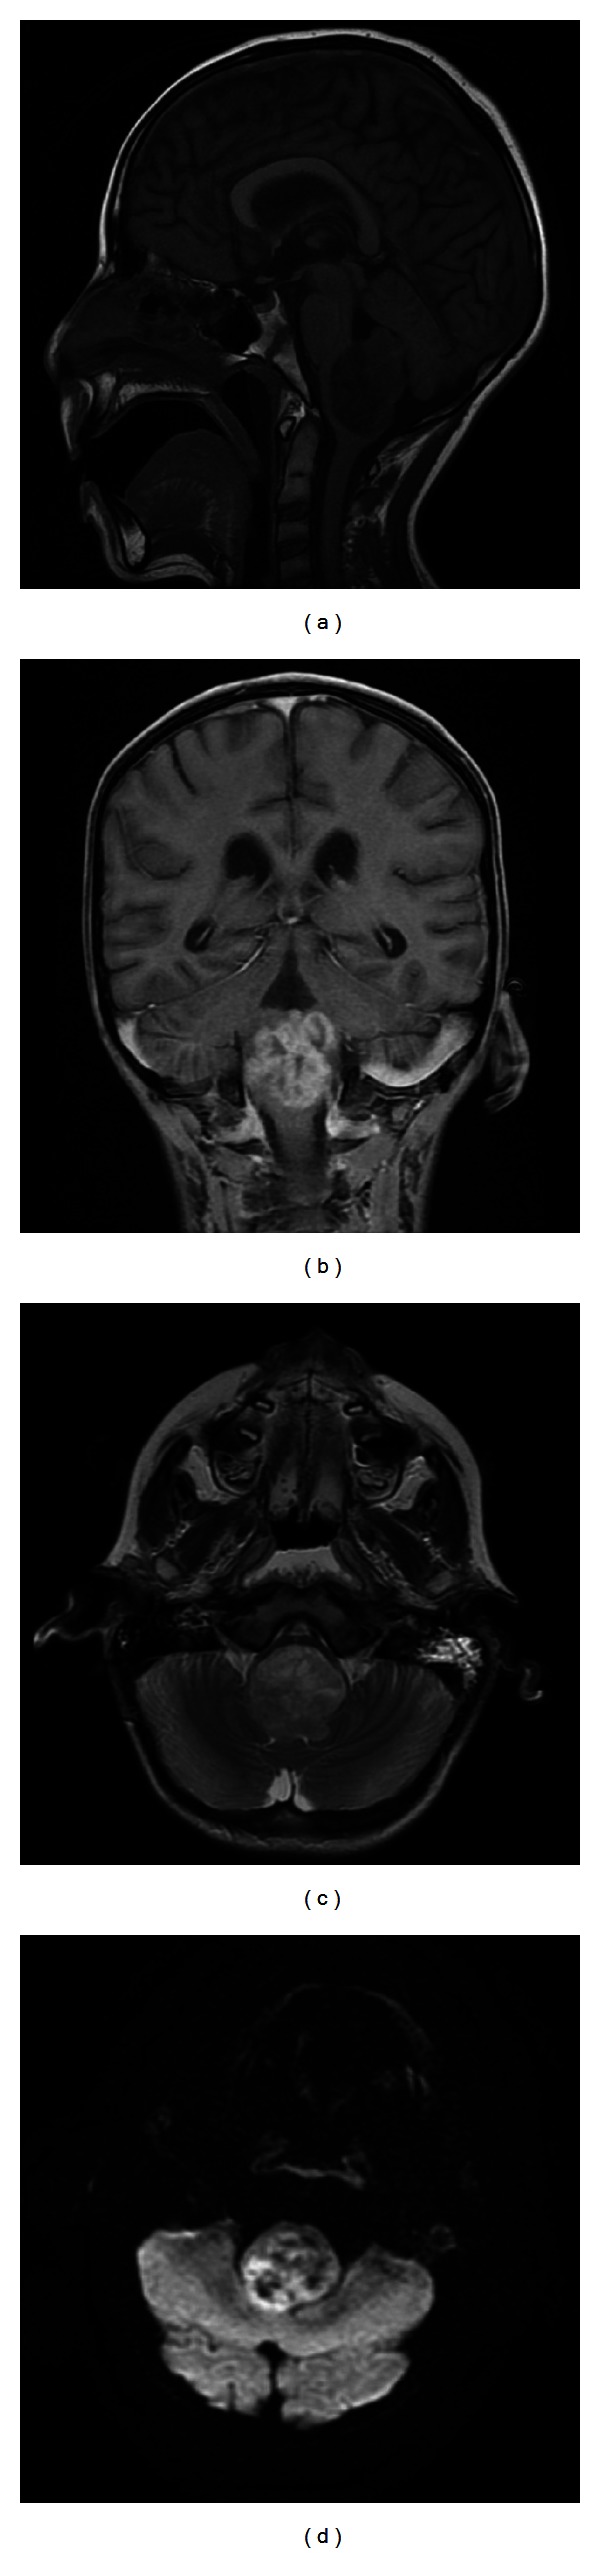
MRI features of Atypical Teratoid Rhabdoid Tumor. Sagittal T1 MRI demonstrates a large heterogeneous dorsally exophytic lower brainstem mass (a) which shows robust enhancement on postcontrast coronal T1 sequence (b). The tumor causes extensive compression of the ventral medulla on transaxial T2 sequence (c) and areas of reduced diffusivity (high signal intensity) on transaxial diffusion-weighted sequence (d).
